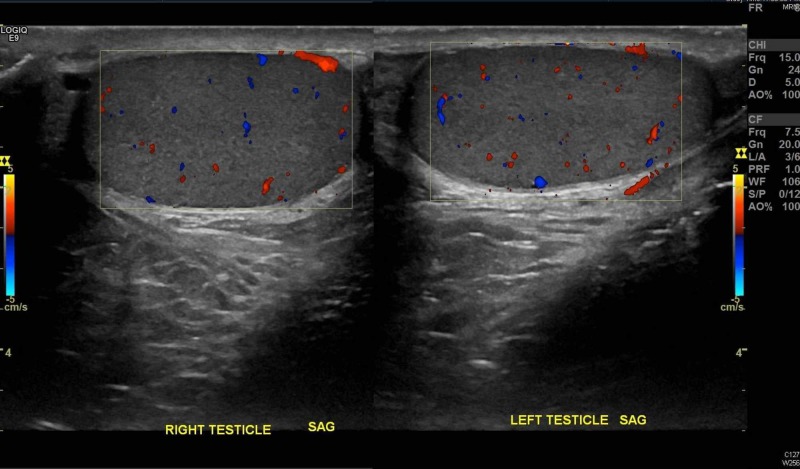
Doppler imaging showing bilateral arterial and venous flow within testes SAG: sagittal view
